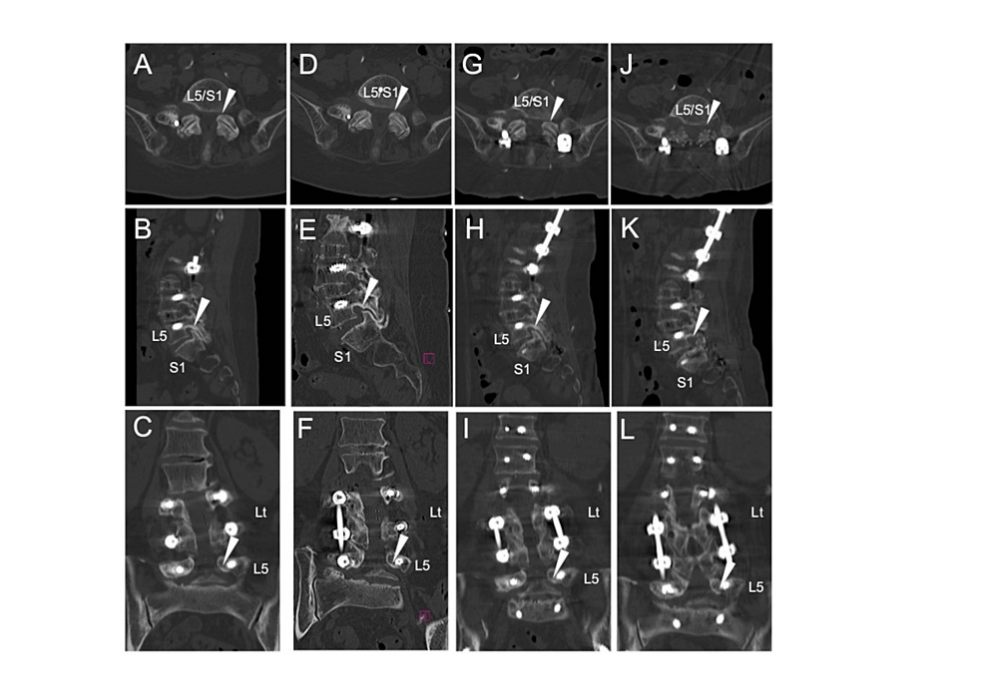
Chronological changes in the right L5/S1 foramen on computed tomography images Following oblique lumbar interbody fusion at the L5/S1, indirect decompression of the same level was temporality achieved (A-F). However, the right L5/S1 foramen (arrowheads) narrowed after posterior fixation surgery (G-I). The stenosis of the L5/S1 foramina was managed with posterior decompression surgery (J-L). Images taken (A-C) before oblique lumbar interbody fusion including the L5/S1 level, (D-F) after oblique lumbar interbody fusion including the L5/S1 level, (G-I) after posterior fixation via percutaneous pedicle screws, (J-L) after posterior decompression of the bilateral L5/S1 foramina. Figures A, D, G, and J show axial images, B, E, H, and K show sagittal images, and C, F, I, and L show coronal images, (Lt: left).
